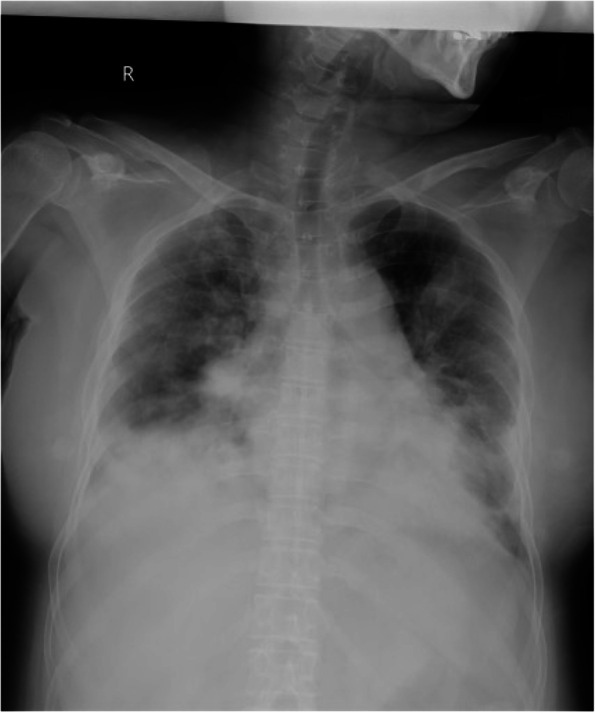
Chest radiography findings show infiltrative shadows on both lung fields
